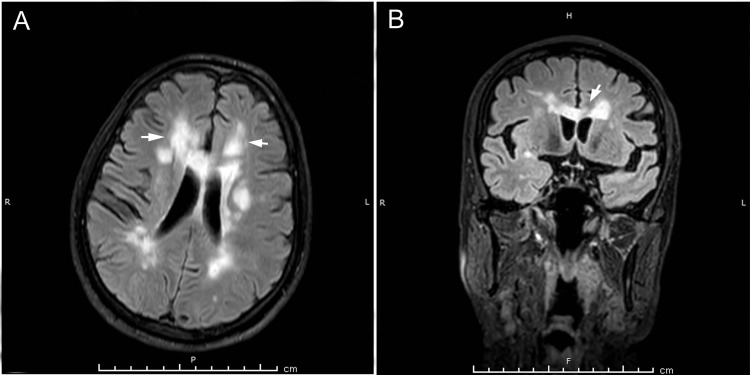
(A) Fluid attenuated inversion recovery (FLAIR) axial scan shows multiple FLAIR hyperintense signal intensities (demarcated by white arrows) in the corona radiata (bilaterally) and in the periventricular regions. (B) FLAIR coronal scan shows multiple FLAIR hyperintense signal intensities (demarcated by a white arrow) in the corona radiata (bilaterally) and in the periventricular regions.
